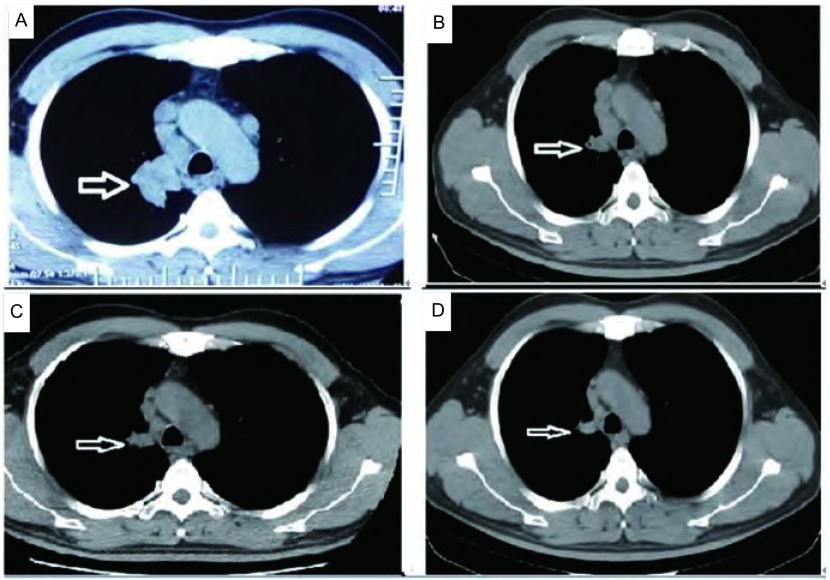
不同时期的肺部CT。A：治疗前肺部CT（2013年4月14日）；B：第2疗程化疗后肺部CT（2013年6月15日）；C：第4疗程化疗后肺部CT（2013年7月17日）；D：应用靶向药物维持治疗30天后肺部CT（2013年8月14日）。图中箭头所指为肺部肿瘤 The pulmonary computed tomography (CT) of different time. A: The pulmonary CT before treatment (April 14, 2013); B: The pulmonary CT after the second course of chemotherapy (June 5, 2013); C: The pulmonary CT after the fourth course of chemotherapy (July 17, 2013); D: The pulmonary CT after applying TKIs 30 days (August 14, 2013). The arrow pointed to pulmonary lesions
